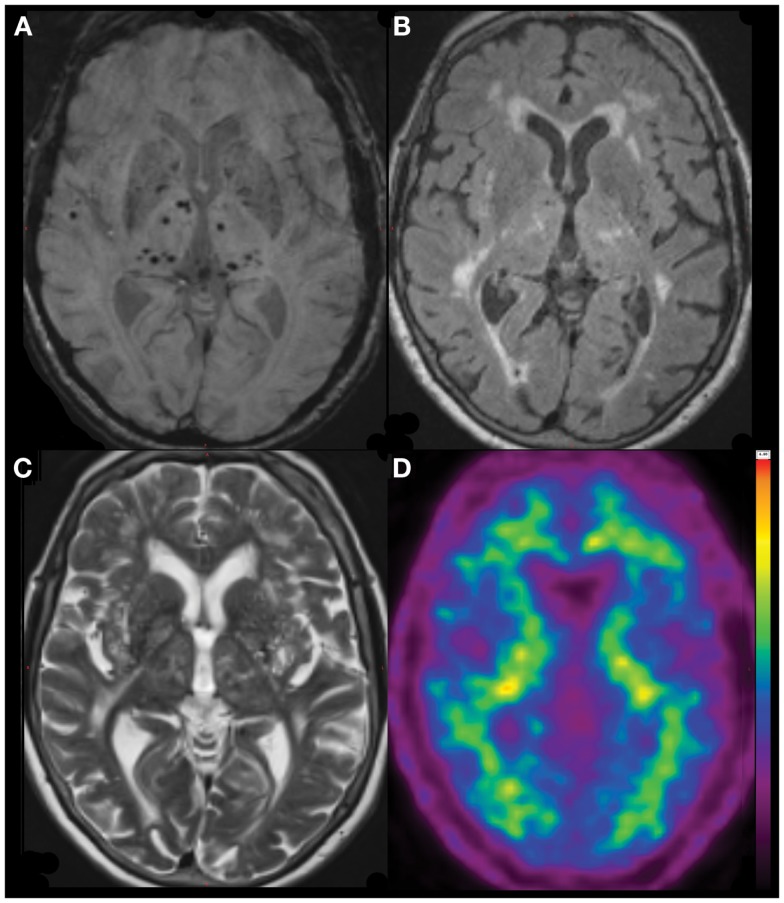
Diffuse (predominantly non-lobar) Microbleeds in an 81-year-old lady referred with AD-type dementia (MMSE 22/30, CDR 1, CDR-SOB 5.5). SWI image (A), with coregistered FLAIR (B), T2 (C) and 11C-PiB PET (D) images demonstrating severe deep white matter hyperintensities but no significant beta-amyloid burden (neocortical SUVR = 1.2), suggesting that the presentation is due to severe cerebral small vessel disease, rather than Alzheimer’s disease.
